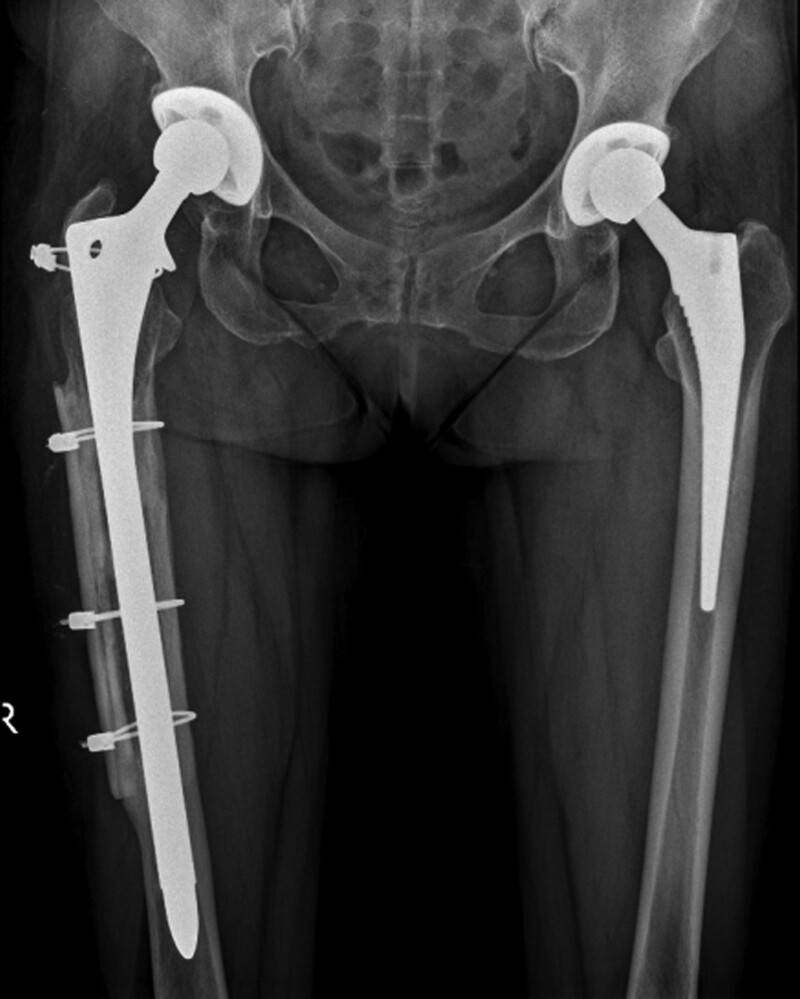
Radiographic analysis 1 year after the second revision of the right hip joint.
